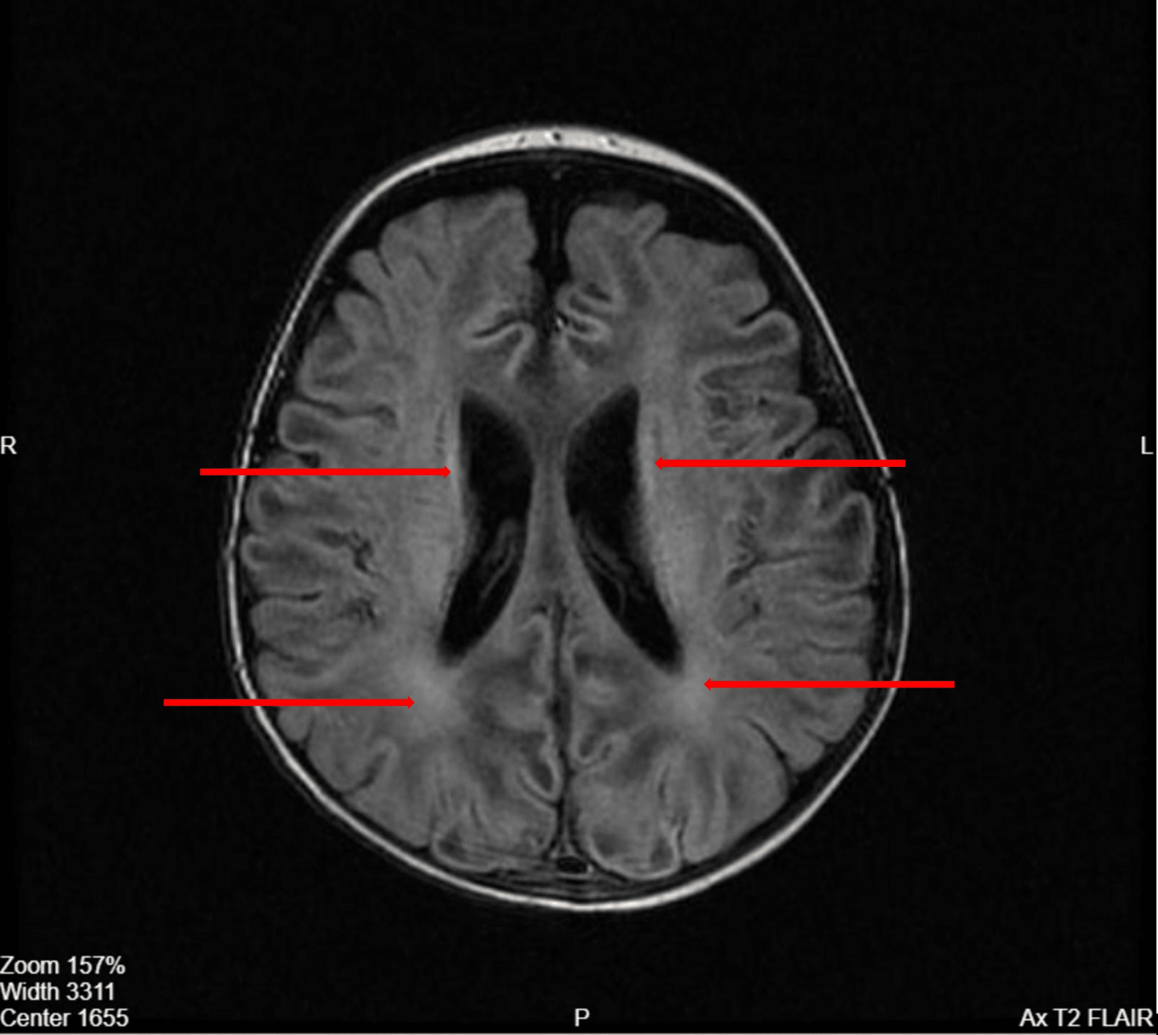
Brain MRI T2 FLAIR sequence showing abnormal signal intensity at the periventricular and occipital white matter on both sides FLAIR: Fluid-attenuated inversion recovery
